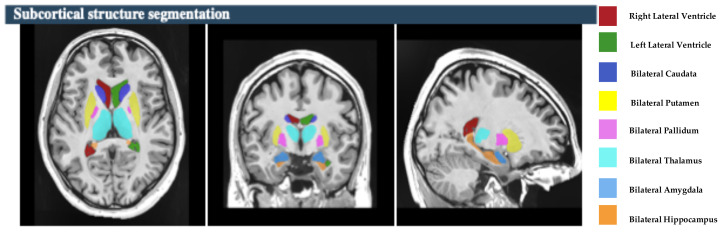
The process of subcortical structure segmentation. Right panel shows the color coded of hippocampal subfields that are overlaid onto the subject’s high-resolution T1-weighted anatomical scan [subject 10].
